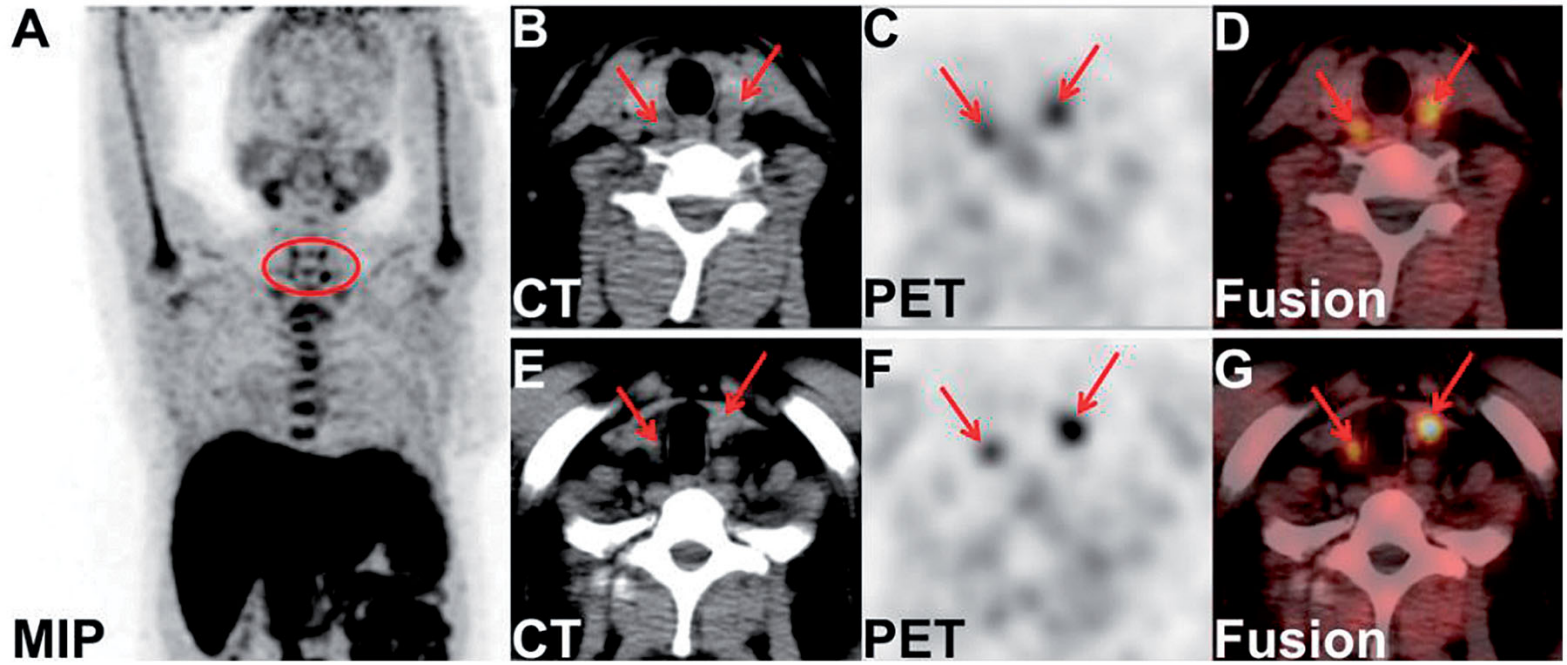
The imaging of parathyroid glands in ^18^F-fluorocholine PET/CT imaging.
